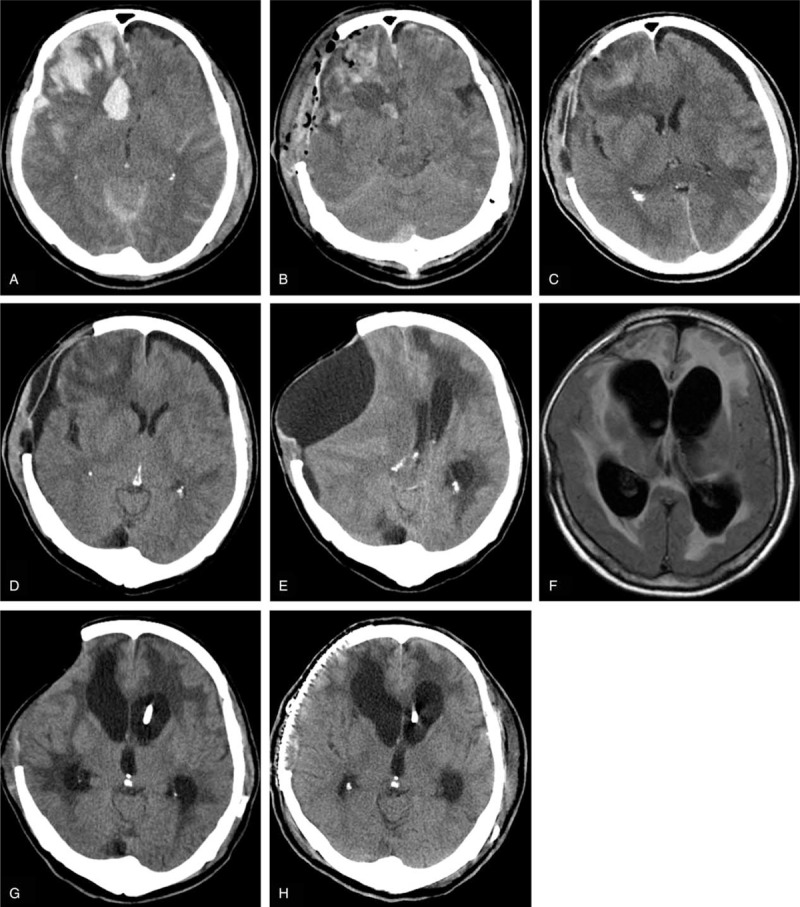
A representative case with paradoxical herniation at 2 weeks to 2 months, showing sinking skin flap. A, CT scan revealing a hemorrhagic contusion in the right frontotemporoparietal region and diffuse brain swelling upon admission. B, CT scan immediately after emergency decompressive craniectomy. Lumbar puncture was performed daily to remove 20 mL CSF per day. C, CT scan on postoperative day (POD) 10, indicating a subdural hygroma in the left frontal region. The patient did not respond to dehydration and lumbar puncture. D, CT scan on POD 30. The patient developed an enlarged subdural hygroma in the left frontal region and a subgaleal fluid collection in the right frontal region after persistent CSF lumbar drainage. E, CT scan on POD 56, showing increased subgaleal fluid accumulation with flattening of overlying cortex and significant midline shift. The GCS was 4. A diagnosis of paradoxical herniation was established, and the external lumbar drainage was weaned. He was placed in the Trendelenburg position and received rapid intravenous hydration. F, MRI on POD 106, showing markedly reduced subdural hygroma and resolution of midline shift. G, Ventricular-peritoneal shunt for hydrocephalus (on POD 122). H, cranioplasty on POD 137. CSF = cerebral spinal fluid, CT = computerized tomography, GCS = Glasgow Coma Scale, ICP = intracranial pressure, MRI = magnetic resonance imaging.
